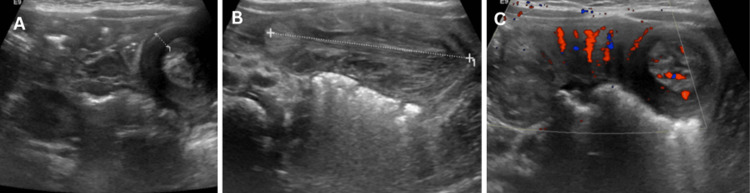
Third episode of ileo-ileal intussusception. Findings are seen unchanged when compared to the previous scan. (A) Target sign with bowel wall edema with a long segment of small bowel intussusception seen extending from the left upper quadrant to the right lower abdominal quadrant, about 2.5 cm in short axis and 6 cm in length. (B) Pseudokidney appearance with mild wall thickening of the involved bowel loops is noted. (C) Blood flow was seen maintained on color Doppler along the layer of the bowel loops and within the surrounding mesentery.
